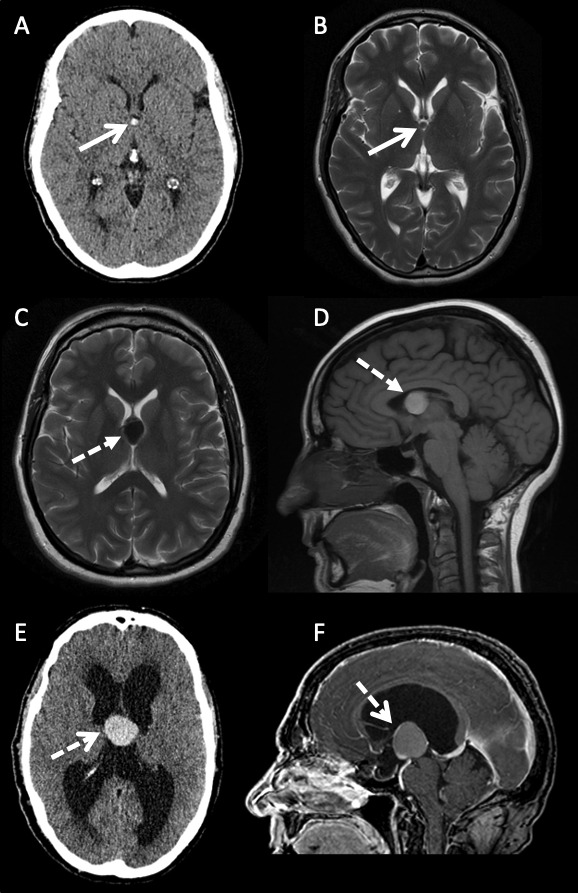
Varying imaging features of colloid cysts. (A) Axial CT scan and (B) axial T2-weighted sequence MRI of a 46-year-old woman with a small CT hyperattenuating (open arrow) colloid cyst within the anterior aspect of the third ventricle. The signal intensity is intermediate to isointense to white matter on the T2-weighted sequence (open arrows). (C) Axial T2-weighted and (D) sagittal T1-weighted MRI sequences of a 50-year-old woman with a large colloid cyst. The lesion is hypointense on T2 and hyperintense on T1 (dashed closed arrow). There is no hydrocephalus. (E) Axial CT and (F) sagittal postgadolinium contrast T1-weighted MRI sequence of a large colloid cyst in a 59-year-old patient presenting with hydrocephalus. The lesion is hyperattenuating on CT, hyperintense on T1 with some peripheral enhancement secondary to displaced and stretched septal veins (dashed open arrows).
